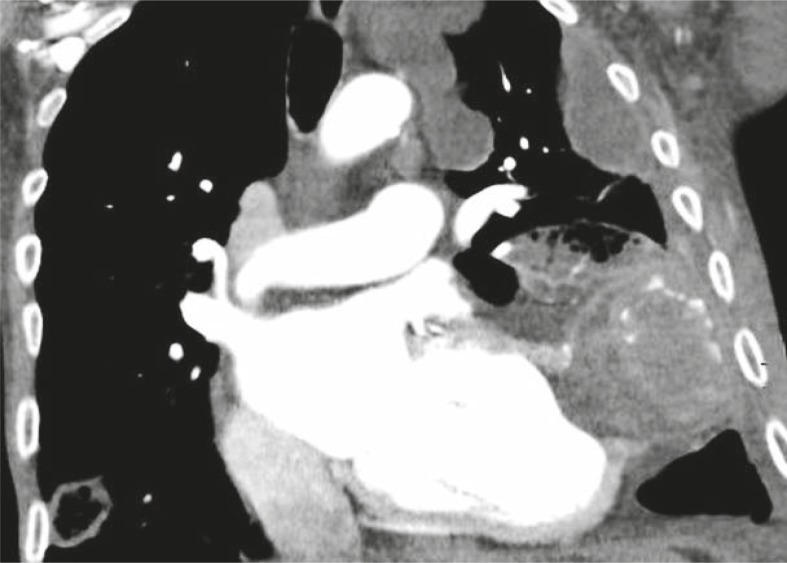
CT image shows a neck created by the orifice of the ruptured myocardium and a large thrombus occupying pseudoaneurysmal cavity.
